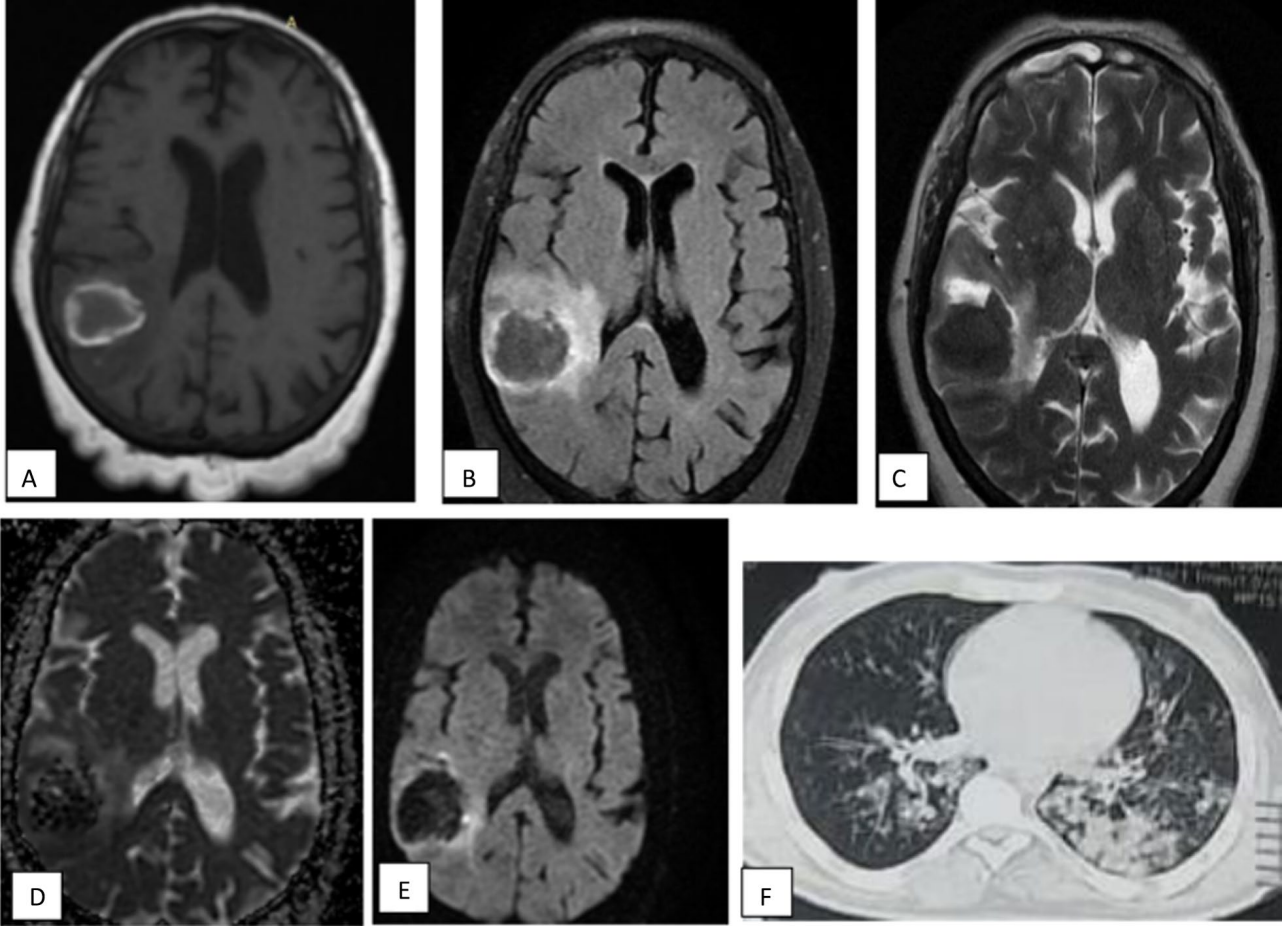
A 50-year-old male presented with sever continuous headache during COVID infection, expressive aphasia. MRI revealed right occipital well defined intra axial space occupying lesion. a Axial T1 WI showed area of peripheral high SI with central area of iso intense signal intensity. b Axial FLAIR show area of peripheral edema (c) axial T2WI showed area of relative low SI with peripheral edema (d) ADC map and (e) DWI show area of peripheral restriction. Finding correlates with subacute intraparenchymal hematoma. f CT lung showed multifocal GGO and consolidation, very typical COVID abnormalities (CORAD-V)
